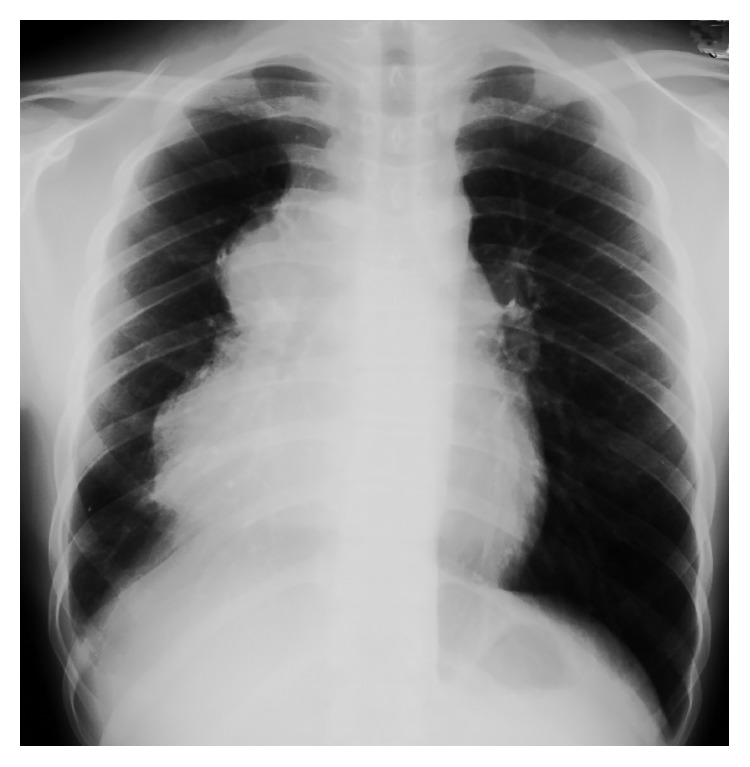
Chest X-ray showing an anterior mediastinum mass.
